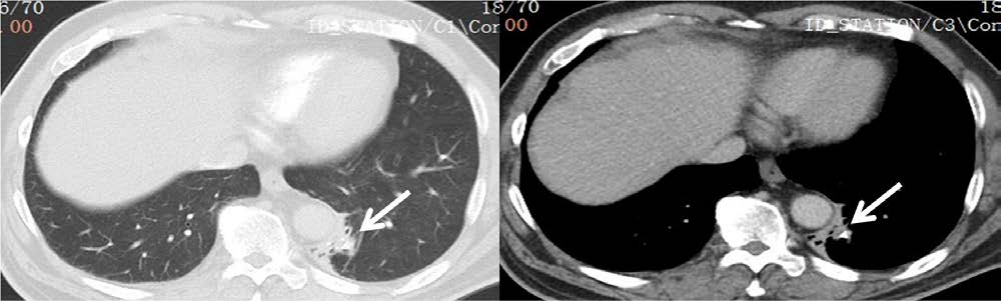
Chest CT upon admission revealed a high-density opacity incarcerated in the distal basal segment of left lower lobe (arrow), along with obstructive pneumonia.
